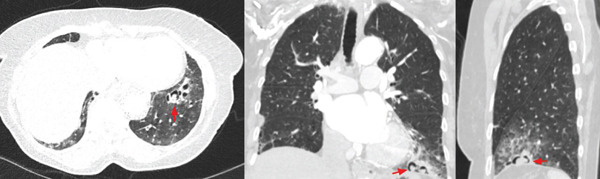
CT chest with diffuse, lower lobe predominant regions of subpleural cystic change, which may be manifestations of rheumatoid‐related or other interstitial lung disease. More focal regions of cystic change visualized at the left lung base with internal solid component (arrow) are seen in axial, coronal, and sagittal cross sections.
